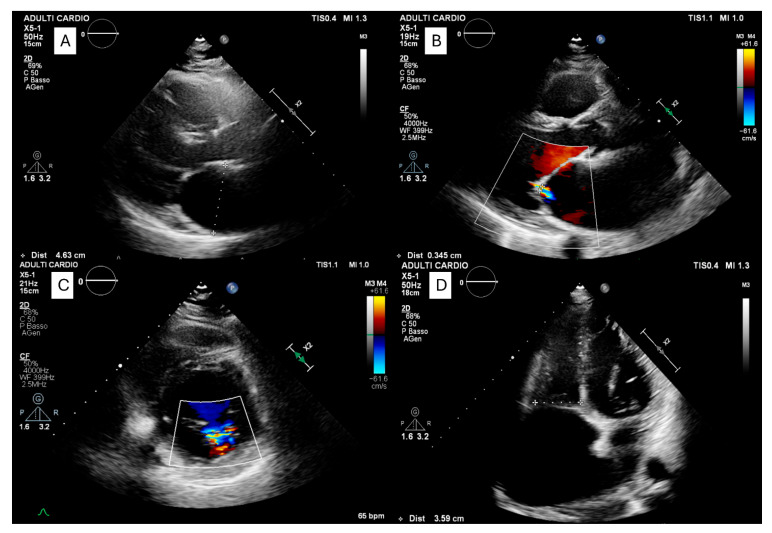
Transthoracic echocardiographic findings in a patient carrying a homozygous variant in the *NPPA* gene. Transthoracic echocardiography showing a parasternal long-axis view (A) with measurement of the left atrial anteroposterior diameter (46 mm). Image (B) demonstrates mild mitral regurgitation with a vena contracta of 3 mm. Panel (C) shows mitral regurgitation on the parasternal short-axis view. In the apical four-chamber view (D) focused on the right heart, severe right atrial enlargement is evident, with preserved right ventricular size.
